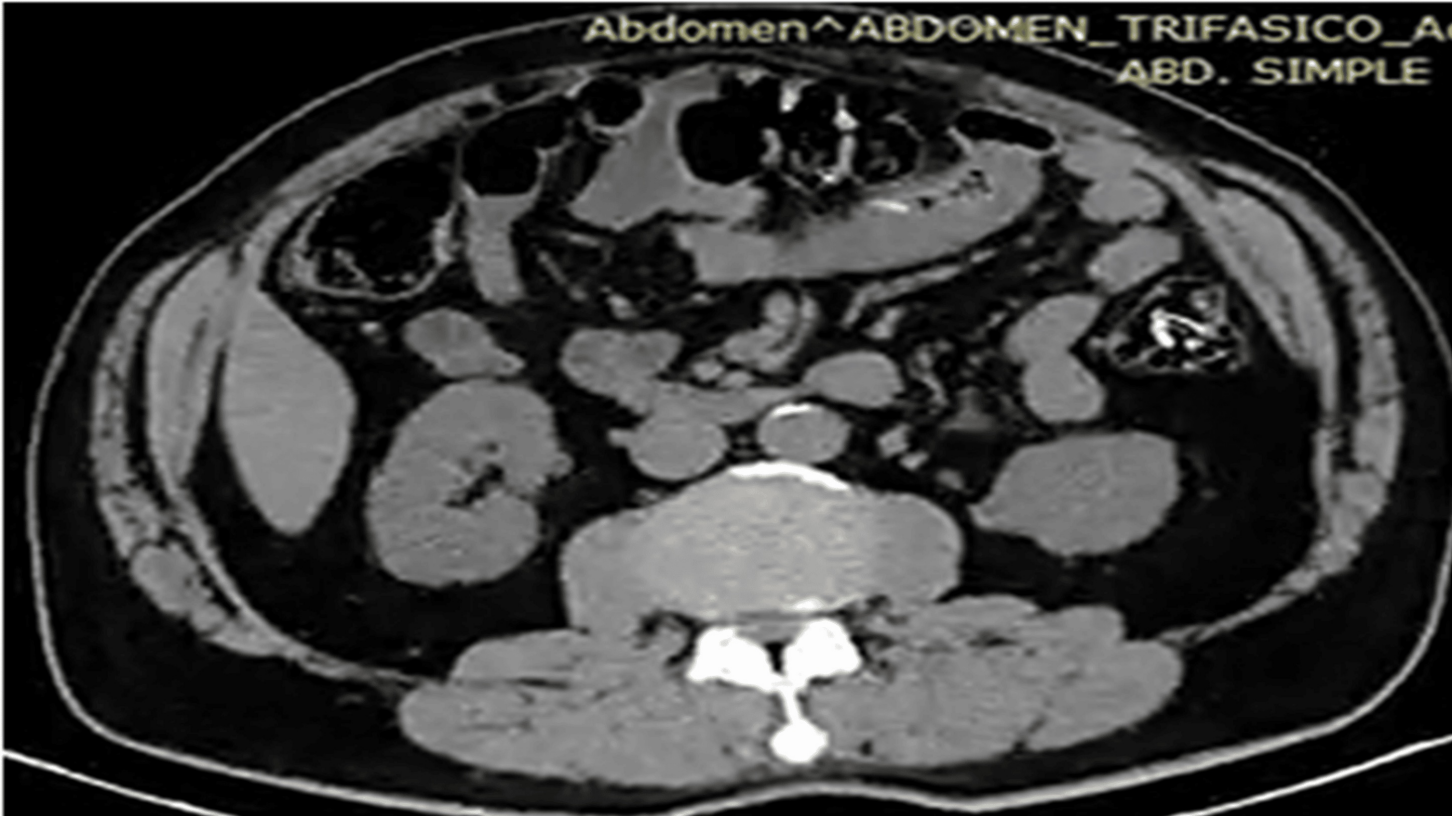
Coronal computed tomography view demonstrating the foreign body oriented perpendicular to the small-bowel axis with localized inflammatory changes
